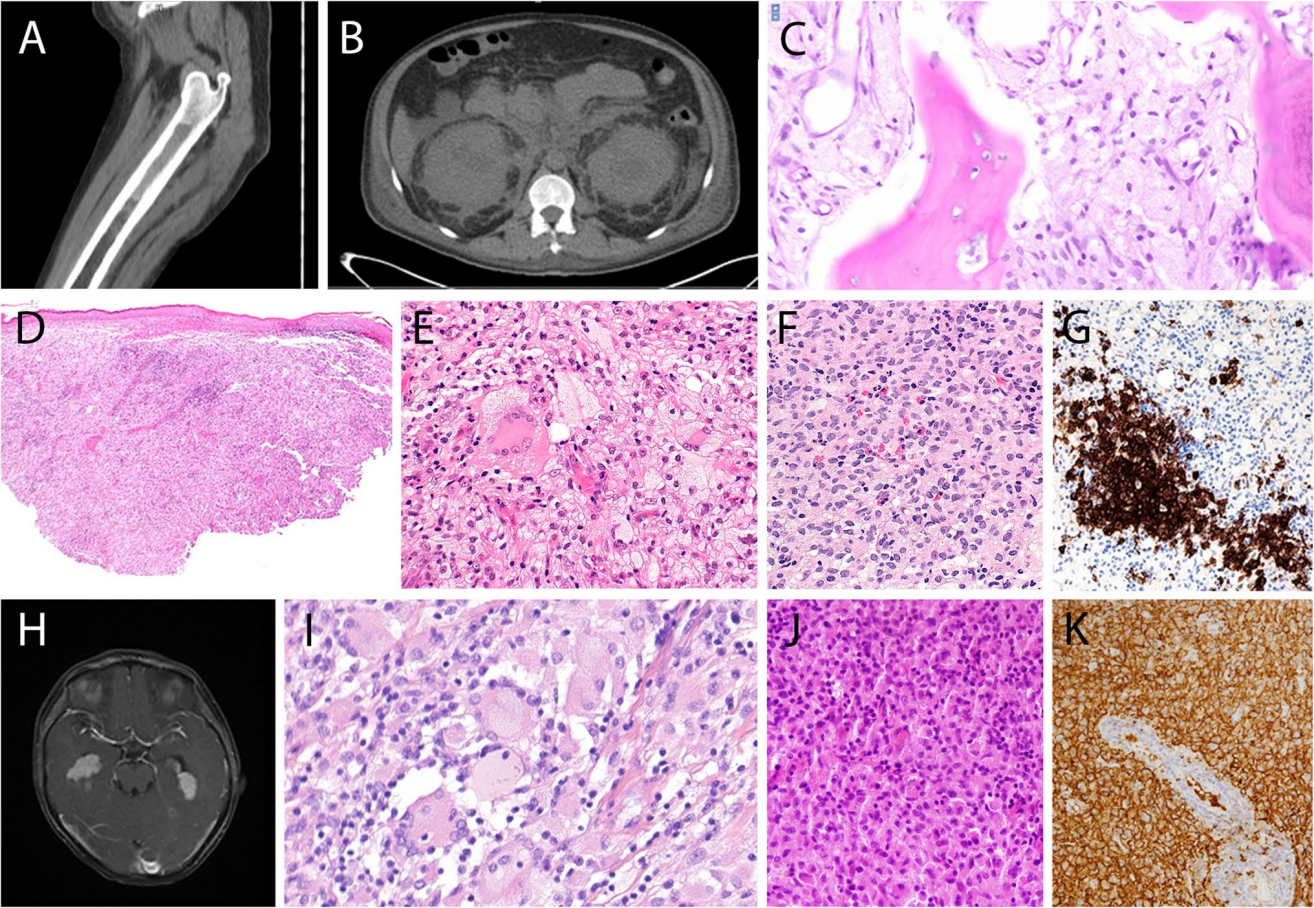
Erdheim Chester disease (ECD), mixed histiocytosis, and systemic “juvenile xanthogranulomatosis” (JXG). A–B Case 221,386 (D. Soliman) ECD. A Typical sclerotic foci in long bone. B CT scan of abdomen with typical “hairy kidney” sign caused by infiltration of perinephric fat. C Case 213,913 (C.L. Cheng), mixed histiocytosis with ECD and LCH. ECD lesion in tibial biopsy with marrow infiltration by foamy histiocytes. D–G Case 221,365 (J. Goodlad), mixed histiocytosis with ECD and LCH. D, E Submammary skin biopsy with foamy histiocytes and occasional Touton giant cells. F LN infiltrate by Langerhans cells with G CD1a expression. BRAF V600E was detected in both lesions. H–K So-called systemic JXG with CNS involvement better classified as non-LC histiocytosis. H, I Case 221,572 (S. Yang). H MRI scan (T1 enhanced) of the brain showing bilateral intraventricular masses. I Proliferation of histiocytes with pale cytoplasm and multinucleated cells, occasional emperipolesis. This case in an 8-year-old male showed a BRAF V600E mutation. J, K Case 221,412 (Z. Fan), 37-year-old male. J Monotonous proliferation of mononuclear histiocytes with K. strong CD163 expression
